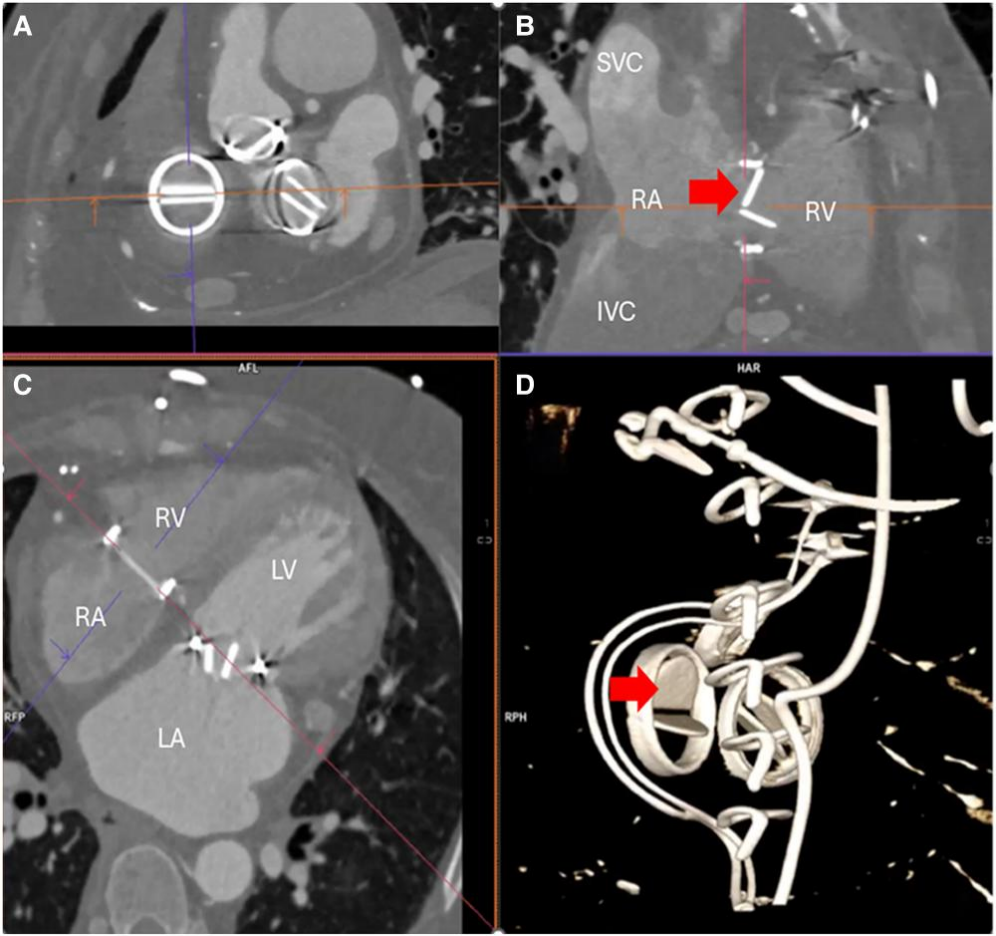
Contrast-enhanced gated cardiac computed tomography after four cycles of ultra-low-dose thrombolysis. The anterior leaflet of the mechanical valve remained immobile in diastole (arrow), and no atrial or valvular thrombus was seen. *Panel A* shows the enface view, and *Panel B* shows the cross-section of the 27 mm St Jude mechanical tricuspid valve. *Panel C* shows the four-chamber view. *Panel D* is the volume rendering showing the immobile anterior leaflet of the tricuspid mechanical valves (arrow), pacing wires, and sternal wires. LV, left ventricle; LA, left atrium.
